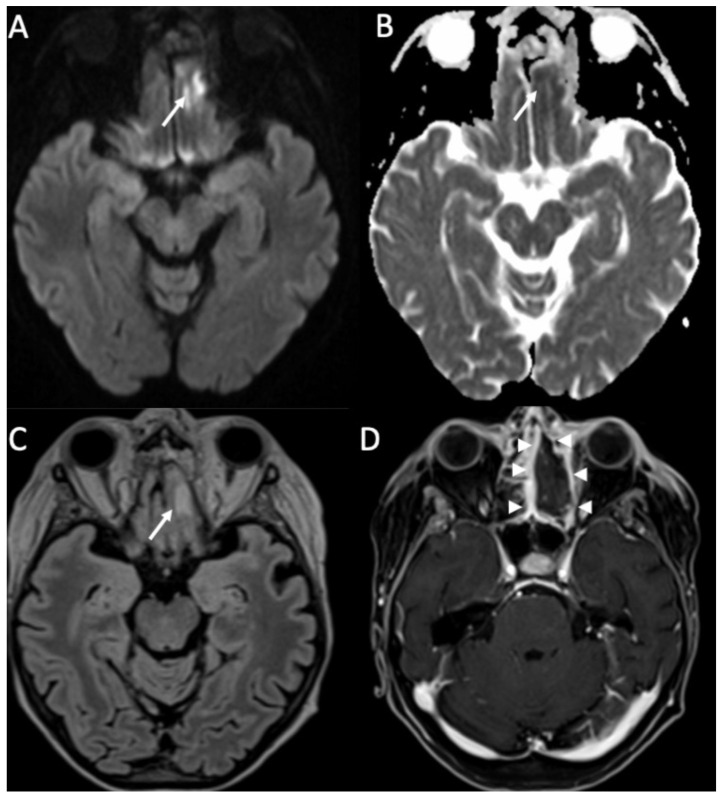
Axial DWI (A), ADC (B), FLAIR (C), and enhanced T1 with fat-suppression depict an area of cerebritis in the left rectus gyrus (arrows in A–C), which shows restricted diffusion and hyperintensity on FLAIR (arrow in C). Also note the sinusitis in the left anterior ethmoidal cells, with necrosis of the mucosa (arrowheads in D).
